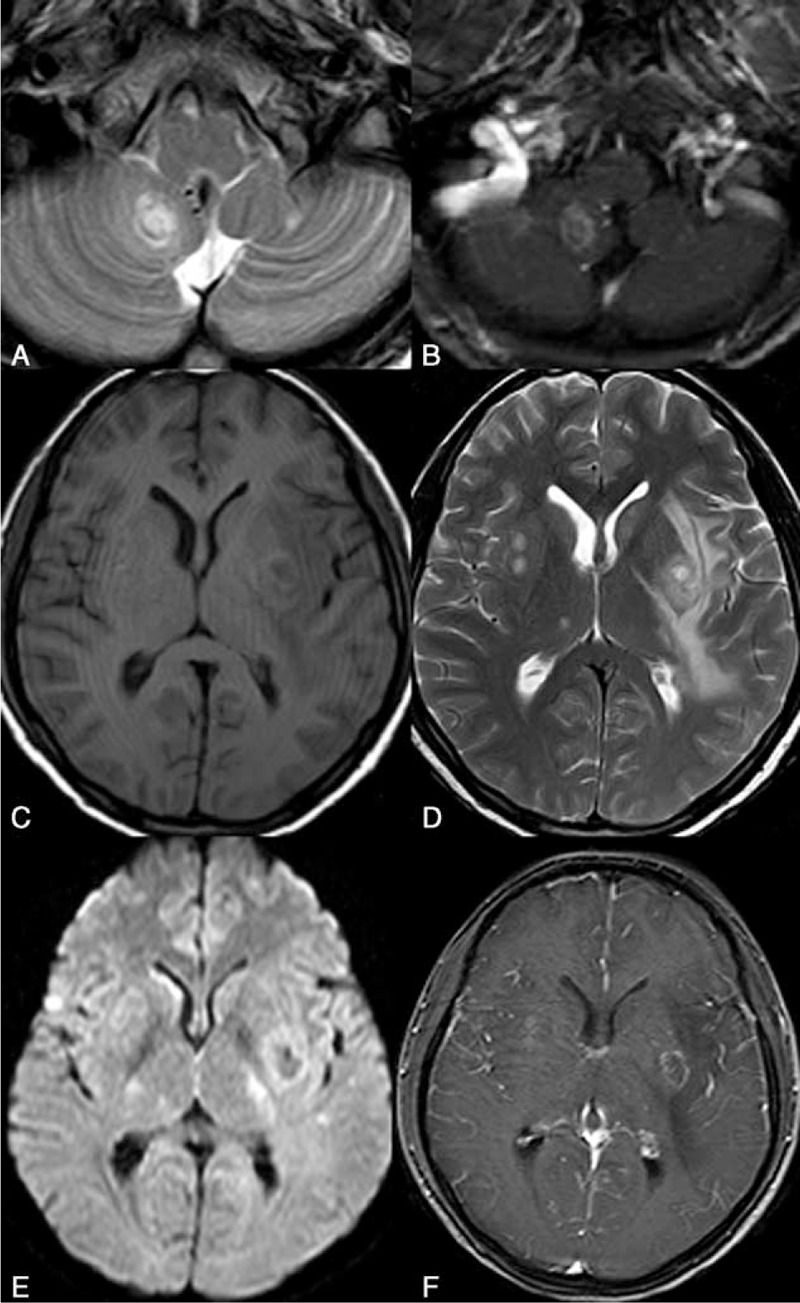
A 25-year-old woman with complaints of headache, fever, and vomiting. Both HIV antibody and cryptococcus were detected and found to be positive in the CSF. Multiple nodular lesions are shown in the right cerebellum and bilateral basal ganglia on MRI. The lesions manifests low intensity on T1WI (C), hyperintensity on T2WI (A, D), no limited diffusion (E), and circular contrast enhancement (D).
